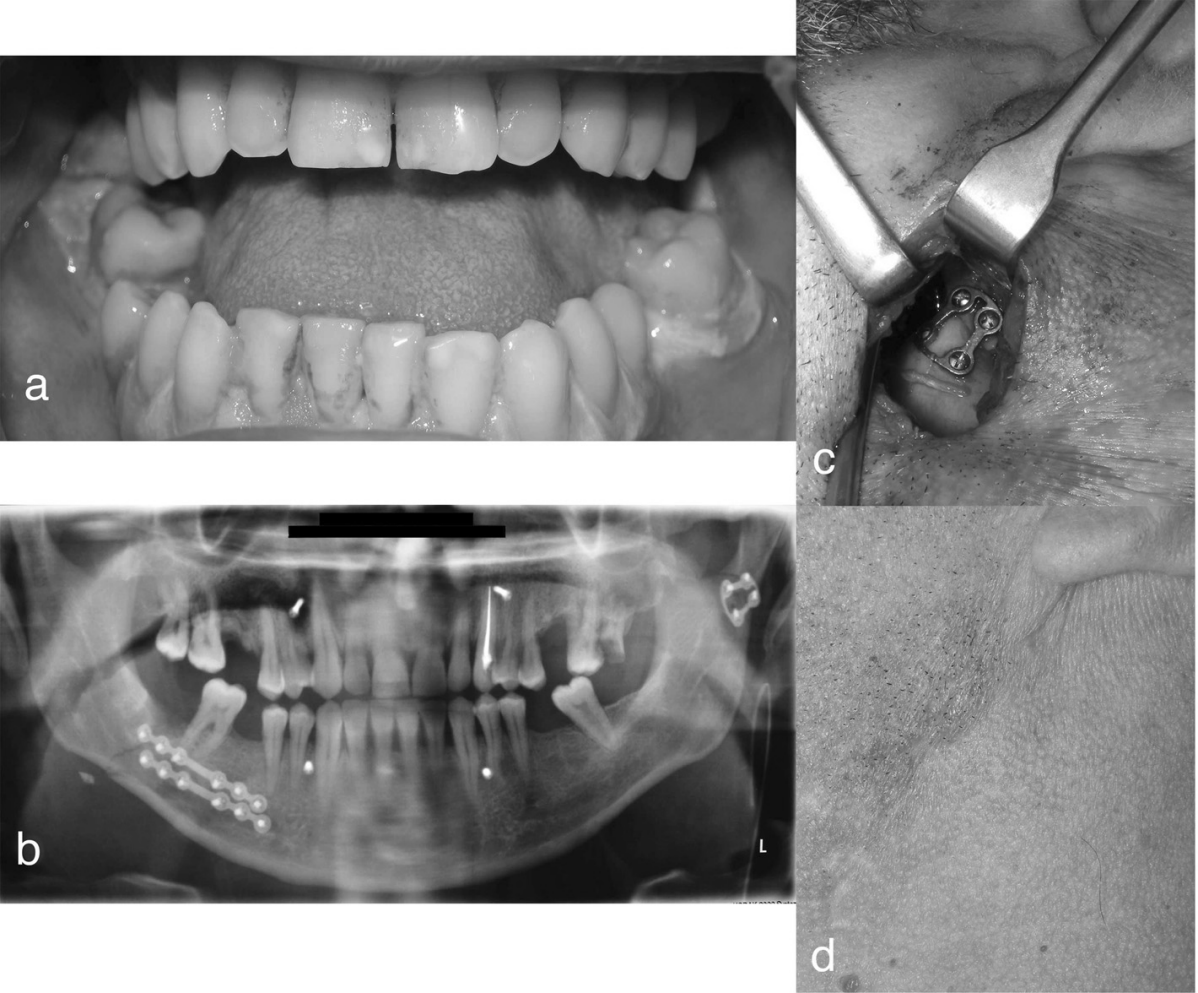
Left mandibular sub-condylar fracture: a) pretrauma mouth opening; b) post-surgery orthopantomography; c) ORIF with a TCP plate; d) scare result.
